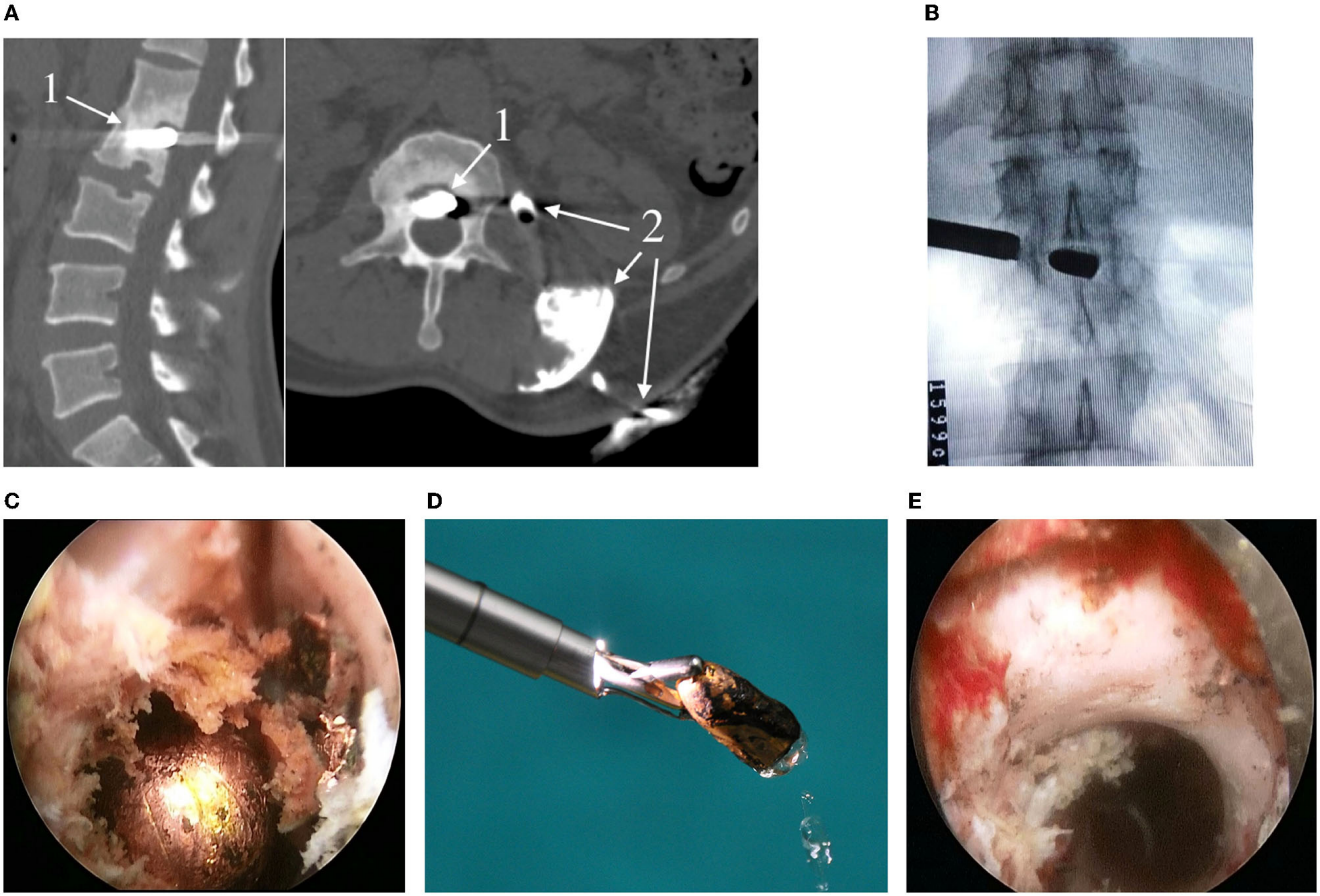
(A) Preoperative sagittal CT (left) and CT fistulography (right) of the lumbar spine: 1—bullet; 2—contrast agent; (B) Radiography of the working port and bullet; (C) Endoscopic stage of the surgery and view of the bullet (described in the text); (D) Capturing and extracting the bullet with forceps (caliber 7.62 mm, length 15 mm); (E) Endoscopic view of the intervertebral foramen after extracting the bullet.
